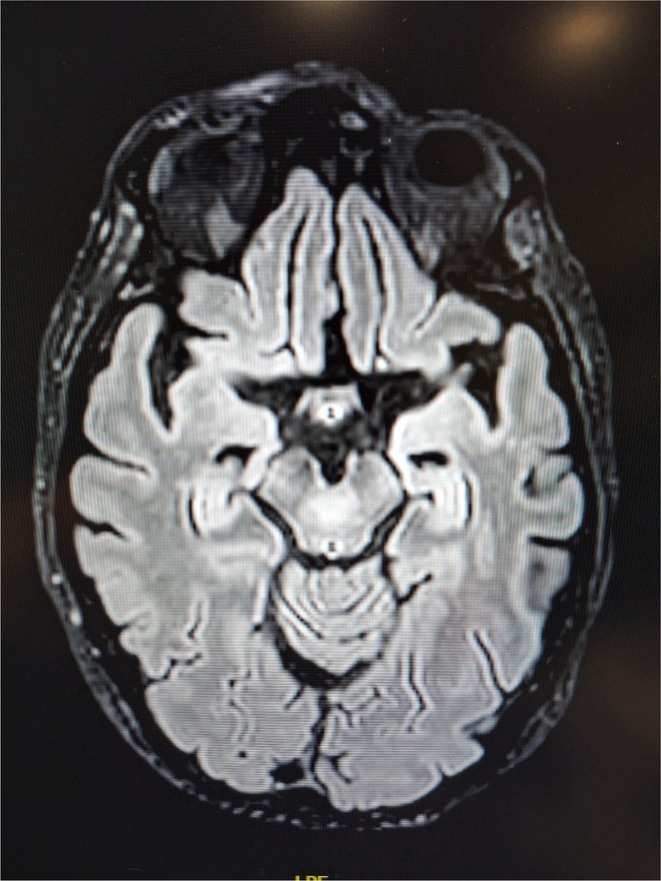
FIGURE 1 Brain MRI of the first patient showed mutiple white matter hyperintensities in T2 and FLAIR in midbrain, pons, both thalami, compatible with IgLON5 encephalitis.
